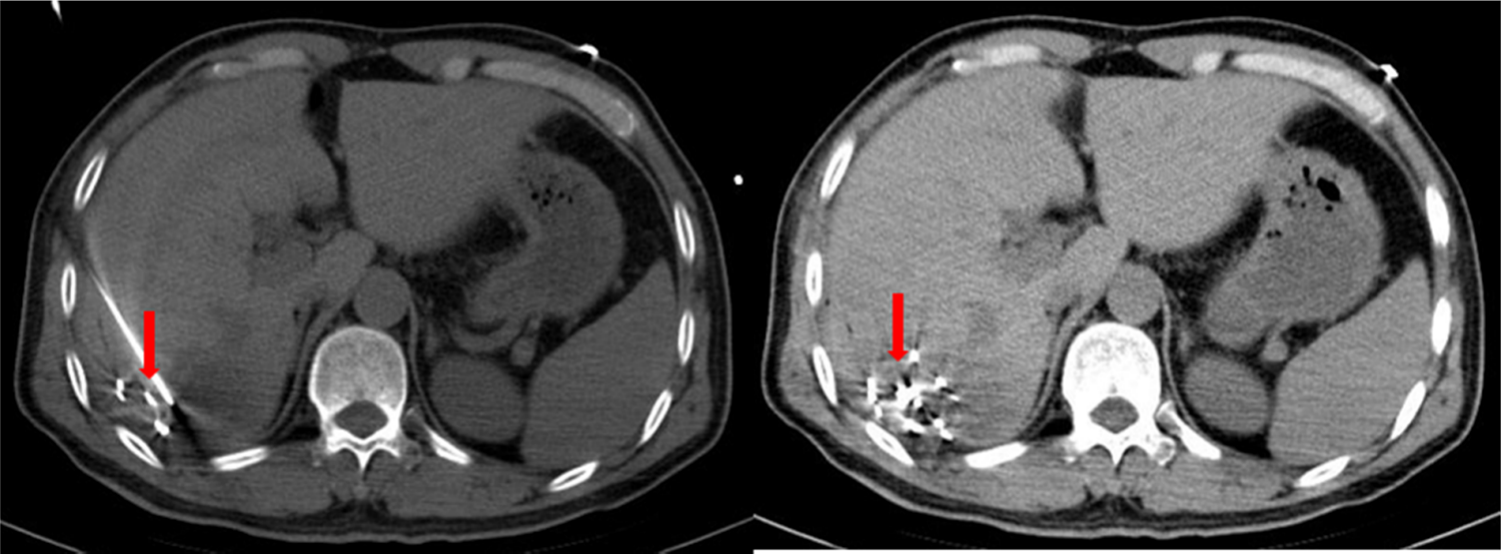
Iodine-125 seed implantation around the lesion in a fan shape with a distance of 1 cm between each two seeds with CT guidance.
